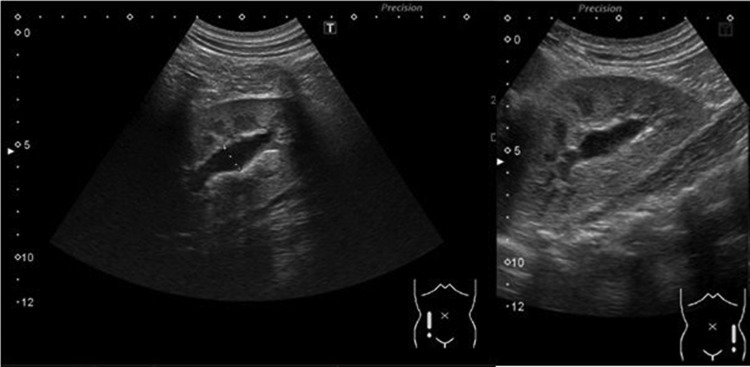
Renal ultrasonography revealed bilateral mild-to-moderate hydronephrosis ∼11 mm after laparoscopic appendectomy on POD10.
